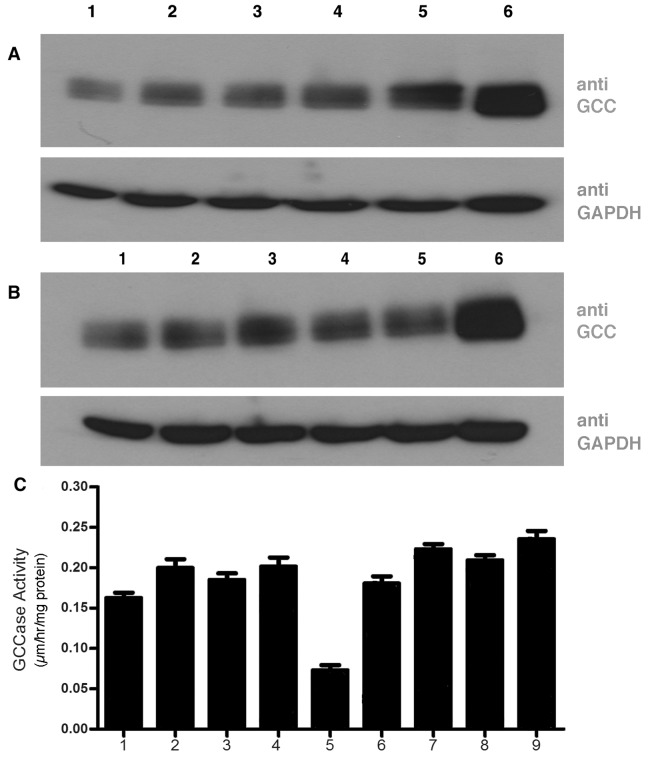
Efficacy of VT and CT constructs to rescue N370S glucocerebrosidase in Gaucher disease fibroblasts Primary skin fibroblasts from an N370S GCC Gaucher disease patient were cultured with ± 260pM toxoid constructs for 4hr and compared to WT fibroblasts. GCC content was determined by western blot (A,B) and enzyme activity(C): panels A (CT treated), B (VT treated): lanes 1- control cells, lanes 2- wt CT/VT, lanes 3- CT0/VT0, lanes 4- CT9L/ VT9L, lanes 5- CT18L/VT16L, lanes 6- normal fibroblast control. Lower panels in A,B = GAPDH western blot loading controls. panel C: GCC activity assayed in triplicate ±SD; lane 1 VT, lane 2-VT0, lane 3-VT9L, lane 4-VT16L, lane 5- untreated control, lane 6-CT, lane 7- CT0, lane 8-CT9L, lane 9-CT18.WT fibroblasts had an activity of 0.9μm/hr/mg protein.
